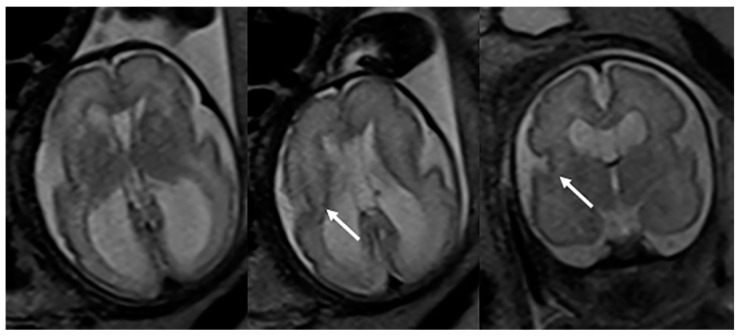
Axial and coronal T2-weighted fetal MRIs during the early third trimester of pregnancy show mild bilateral perisylvian polymicrogyria (arrows) and ventriculomegaly compatible with Zellweger syndrome (confirmed after delivery).
